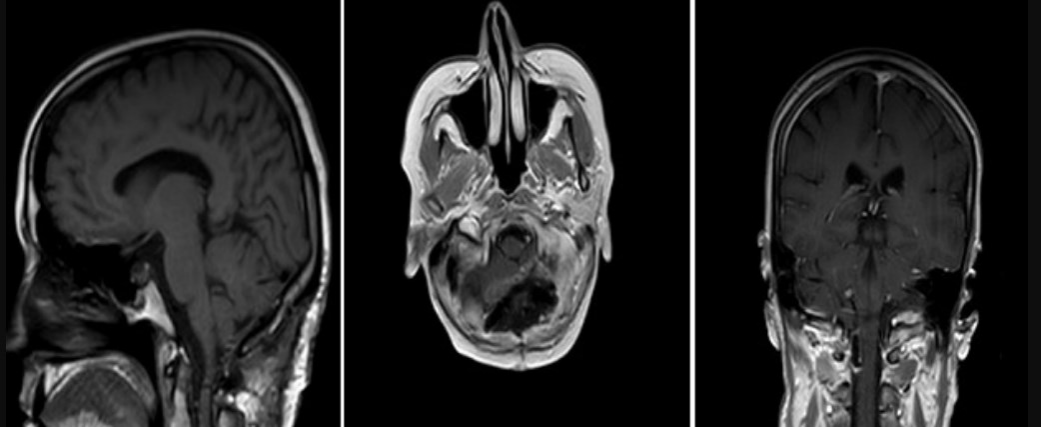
54 years old female patient post-op MRI images
